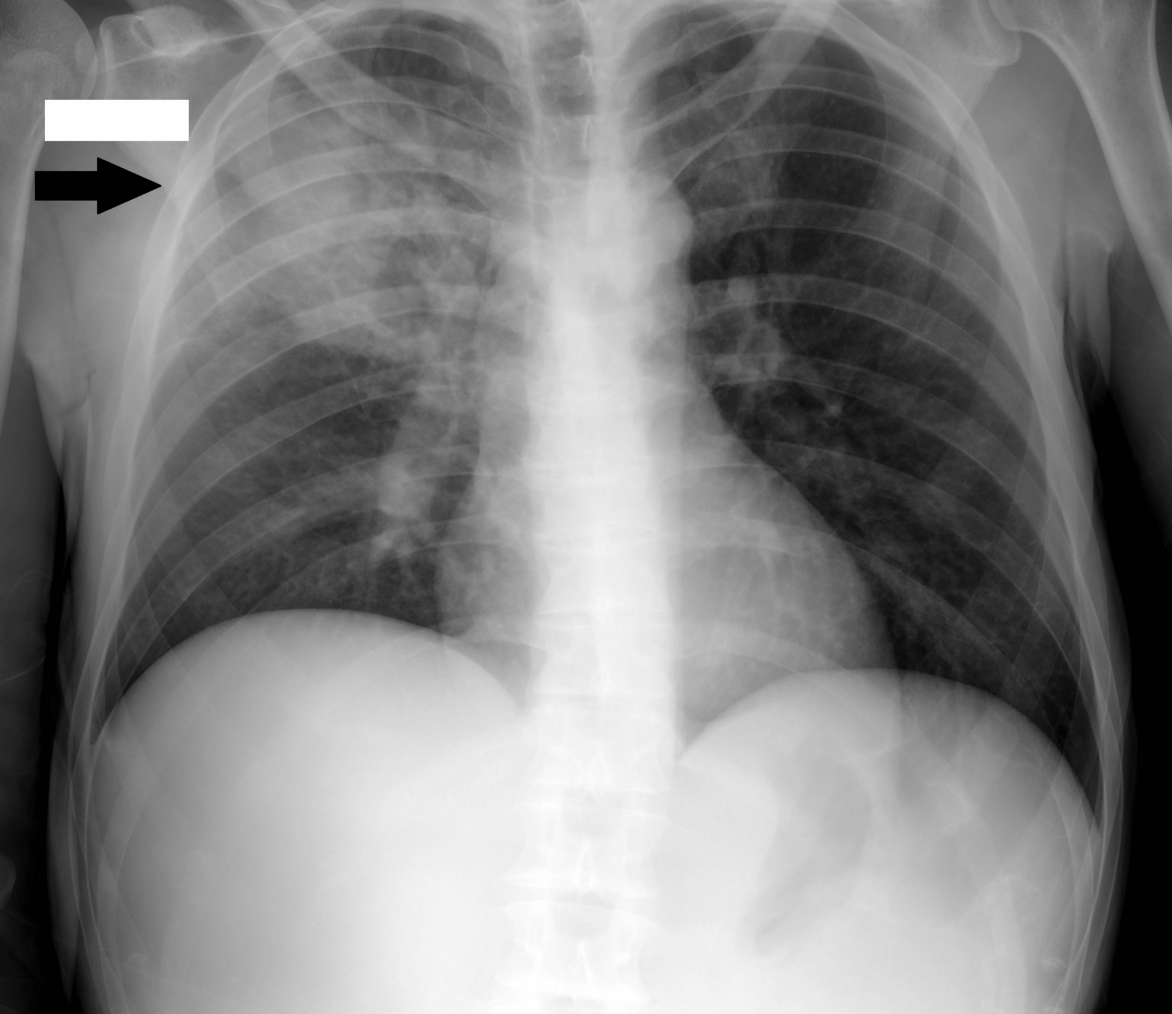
Chest radiography showing a large opacity in the right upper pulmonary field.
